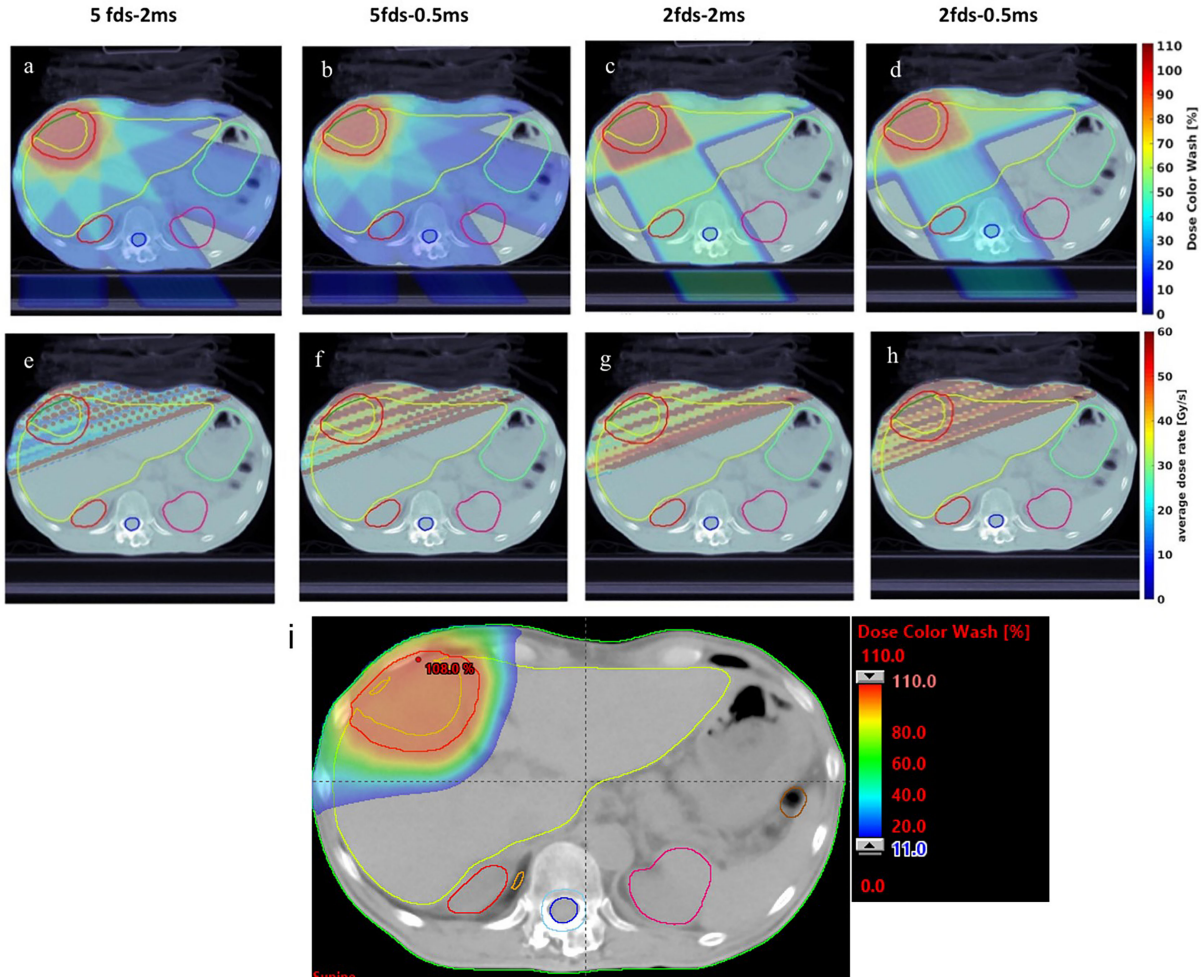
One example of the dose distribution for a single fraction: (A) 5 fields—2 ms MST, (B) 5 fields—0.5 ms, (C) 2 fields—2 ms, and (D) 2 fields—0.5 ms liver transmission plans, overlaid the CT images. The dose rate distribution for a single fraction: (E) 5 fields—2 ms MST, (F) 5 fields—0.5 ms, (G) 2 fields—2 ms, and (H) 2 fields—0.5 ms liver transmission plans, overlaid the CT images. (I) The 2D dose distribution of the SBRT plan for the same patient.
